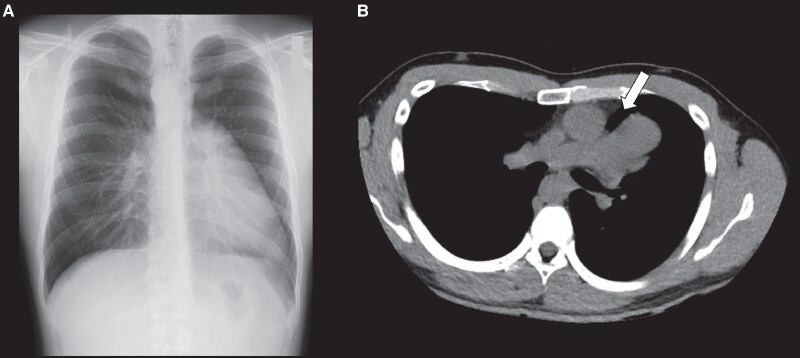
Chest radiography and computed tomography of a 29-year-old man. Chest radiograph showing a leftward shift of the cardiac silhouette with no tracheal deviation, prominent pulmonary arteries, and interposed lung tissue, causing a radiolucent zone between the heart and the diaphragm (*A*). Computed tomography showing interposition of the pulmonary tissue between the aorta and main pulmonary artery (white arrow), indicating the presence of a congenital pericardial defect (*B*).
